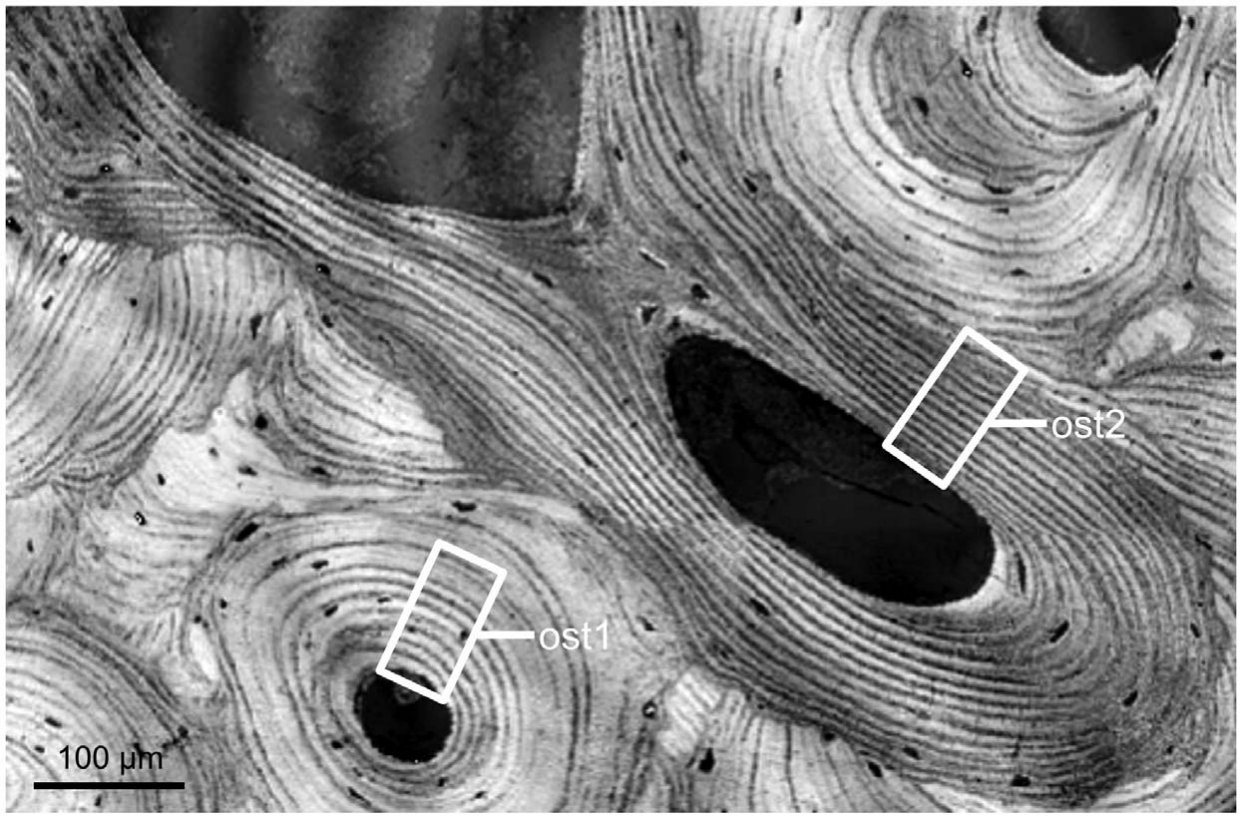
SAM image (amplitude of the reflected ultrasound beam coded in gray levels). The data analysis was performed on an identical site-matched area on each one of the two investigated osteons (namely *ost1* and *ost2*).
